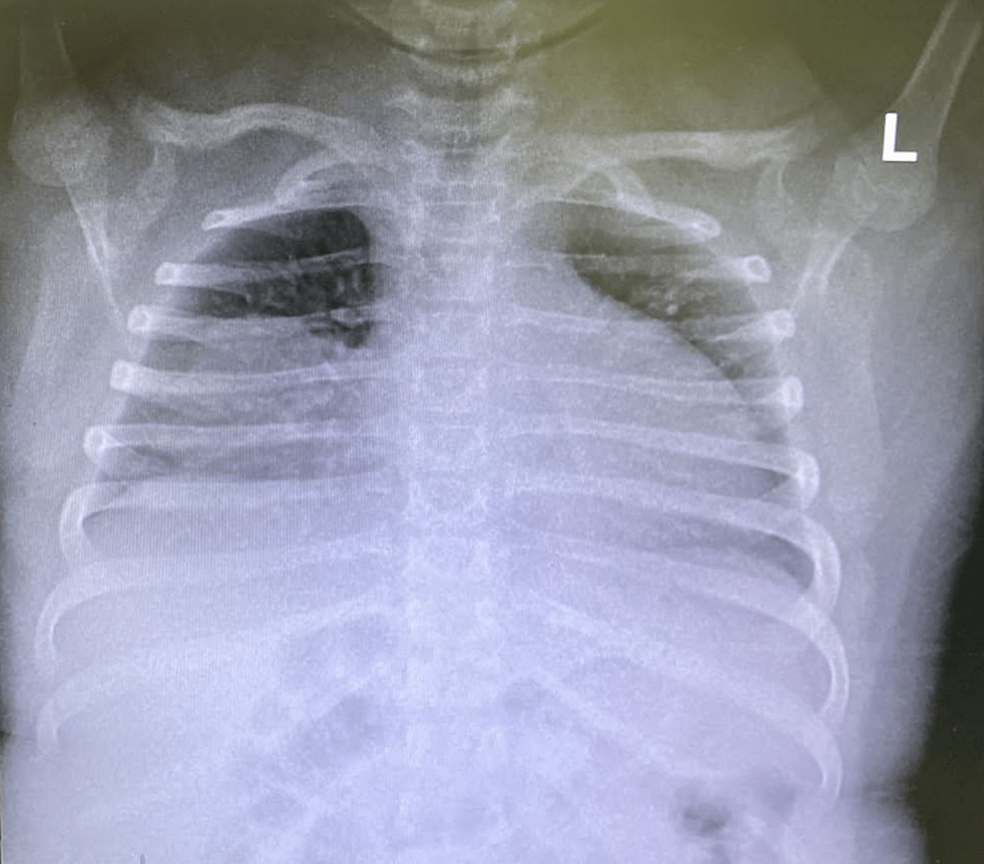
Chest X-ray showing hyperinflated lungs and a mass on the right side of the chest extending to the abdomen.
